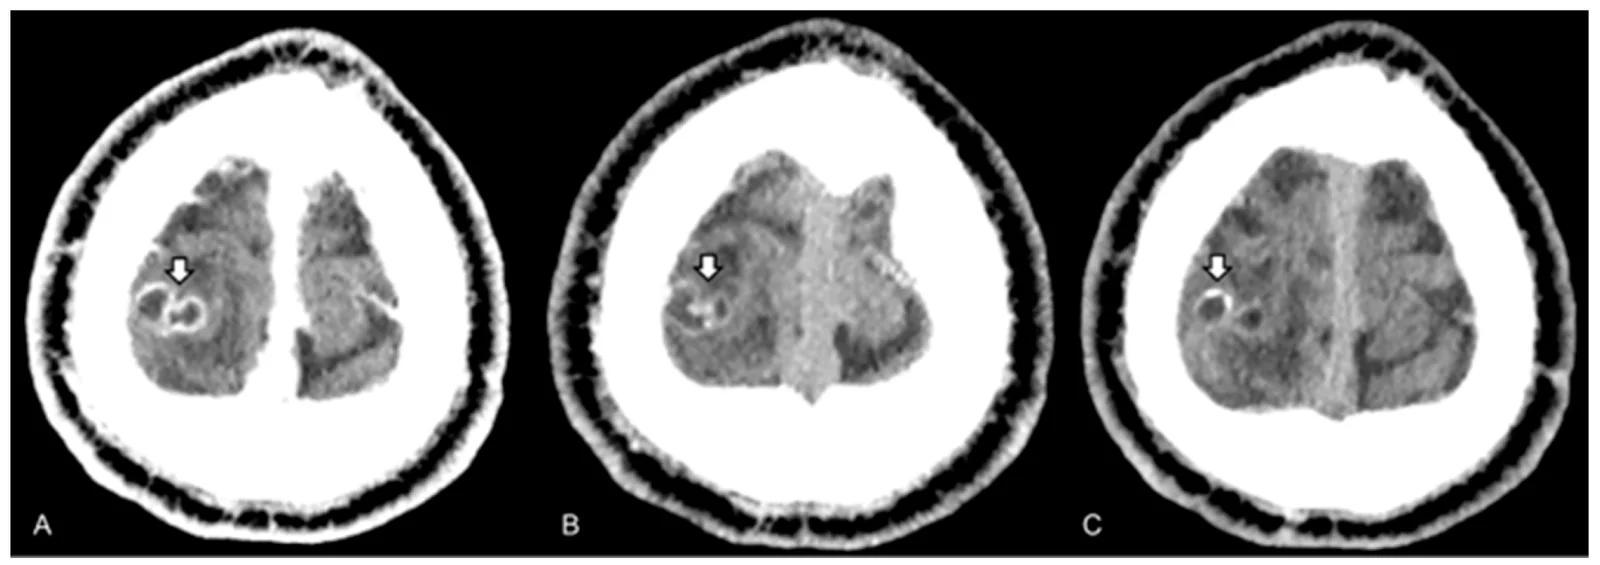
(A) Axial contrast-enhanced CT image demonstrates a multilobulated cystic lesion in the right parietal region with peripheral ring-like enhancement (arrow). (B,C) Axial unenhanced CT images at corresponding (B) and slightly higher (C) levels demonstrate thin peripheral calcification along the lesion wall (arrows). There is no hyperdensity to indicate gross intralesional haemorrhage. Hypodensity of the surrounding parenchyma indicates vasogenic oedema.
